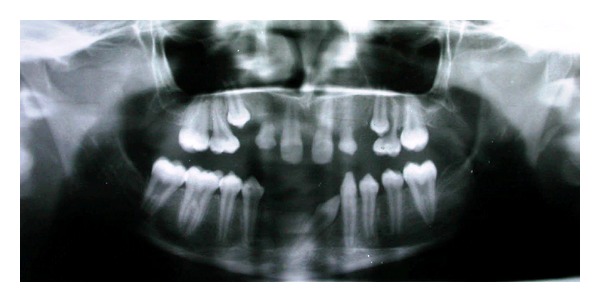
Orthopantomograph showing multiple dental anomalies.
